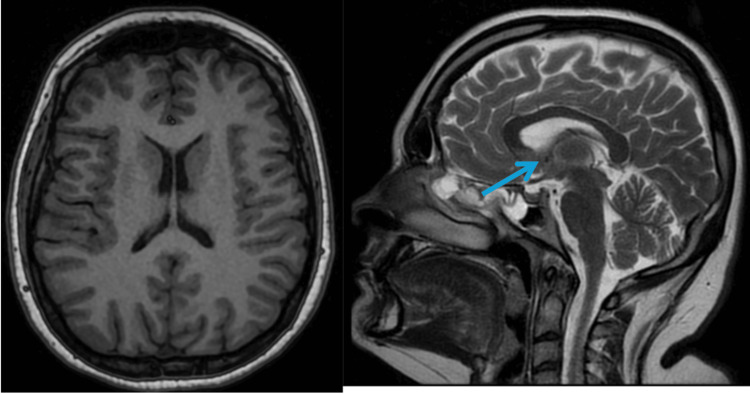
MRI Brain - Cross-sectional & Sagittal Views Reported as normal.
